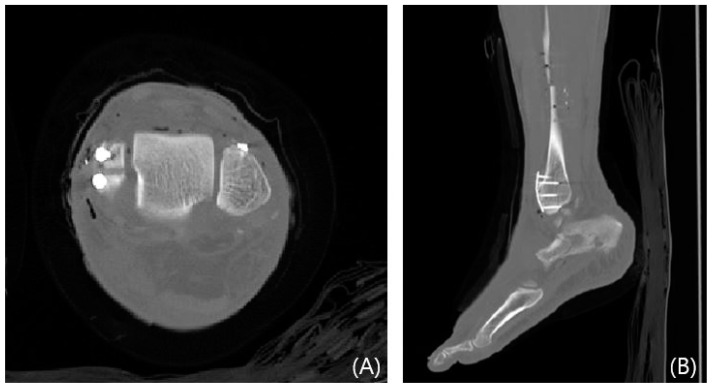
Postoperative CT images showing mini-plate fixation for a Wagstaffe tubercle fracture. Axial (A) and sagittal (B) views.
